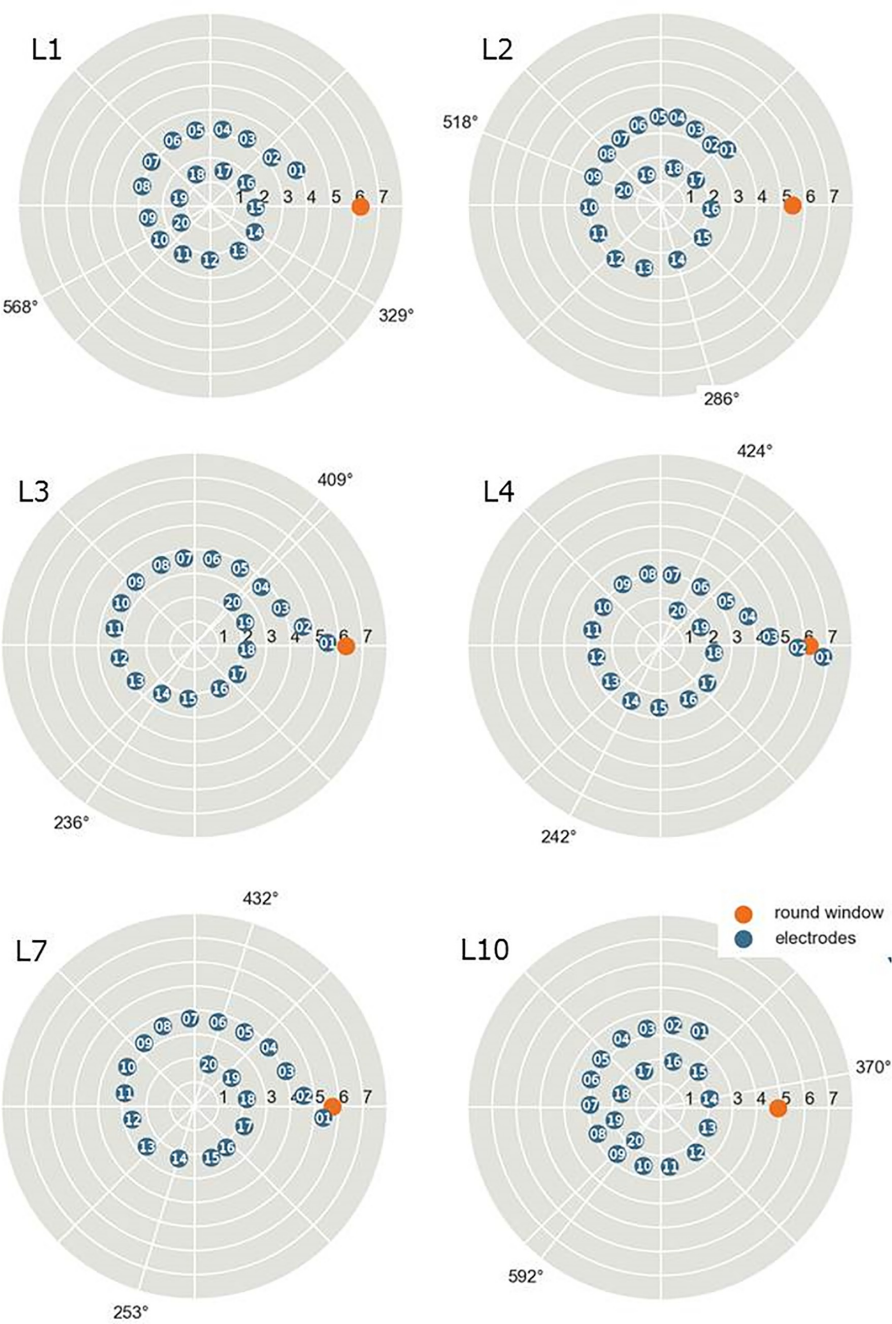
Electrode positions for 6 listeners based on CT scans just after surgery. The two numbers indicate the insertion angle of electrodes 14 and 20.
